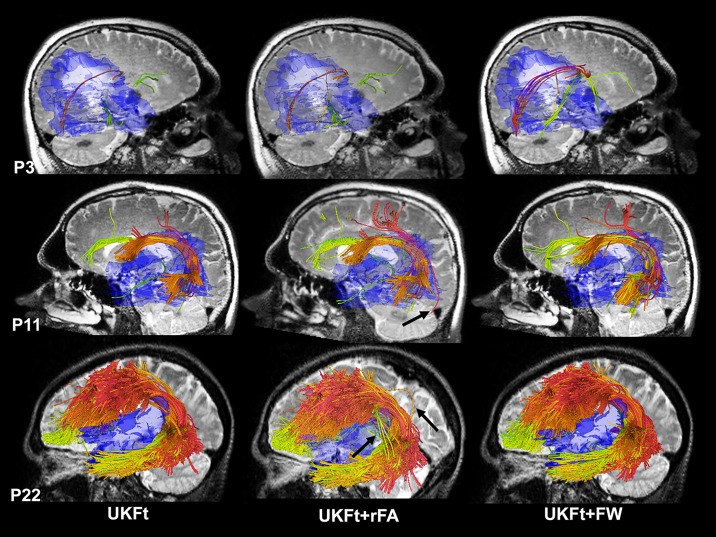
Automatically detected arcuate fasciculus tract clusters in example datasets from patients with edema. Views are from left or right based on the involvement with edema, using the UKFt, UKFt+rFA and UKFt+FW methods of P3, P11, P22. A T2-weighted image is shown behind the fiber tracts. Edema is shown in transparent blue. Tract colors indicate individual fiber clusters as defined in the atlas. For the UKFt+rFA method, the FA thresholds were set to 0.03, 0.07 and 0.07 for the three cases, respectively, to achieve the most similar AF volumes to those obtained using the UKF+FW method. Overall, reducing the FA threshold and adding the FW model both resulted in visually larger AF tracts on the three cases compared to UKFt. In P3, the UKFt+FW method obtained more visually apparent anatomically correct AF fibers (red). In P11 and P22, while tracts obtained using the UFKt+rFA and UKFt+FW methods are visually similar, the UKFt+rFA method introduced more visually apparent false positive fibers (as indicated by the black arrows) than the UKFt+FW method.
